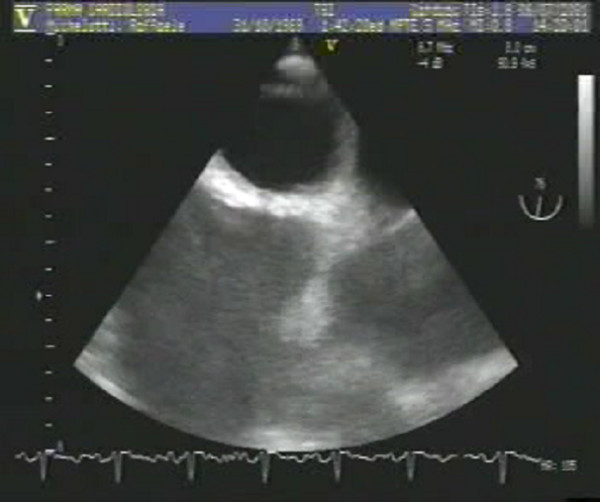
Transesophageal echocardiogram (TEE transverse view 70°). Bulky and serpiginous formation referring to a thrombus that is laid through the foramen ovale and which protrudes in both atria, in burden cycles overcoming the right atrium-ventricular plane.
